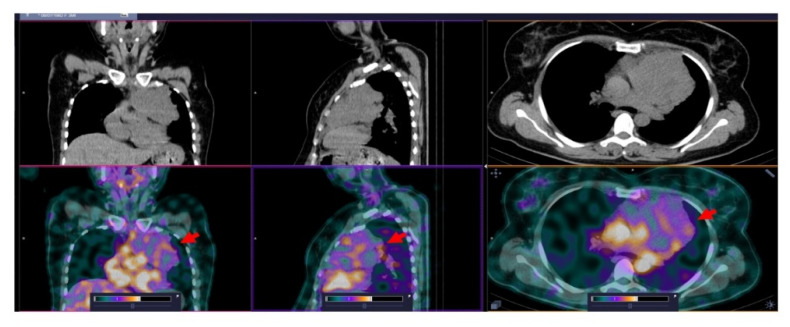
SPECT/CT images of patient 3 at 2 h after injection of [^99m^Tc]Tc-TG. Elevated [^99m^Tc]Tc-TG uptake in the mediastinal tumor (SUVmax = 1.3) is visualized (arrows). The upper setting of the scale window (60% of the maximum number) was adjusted to visualize the lesion.
